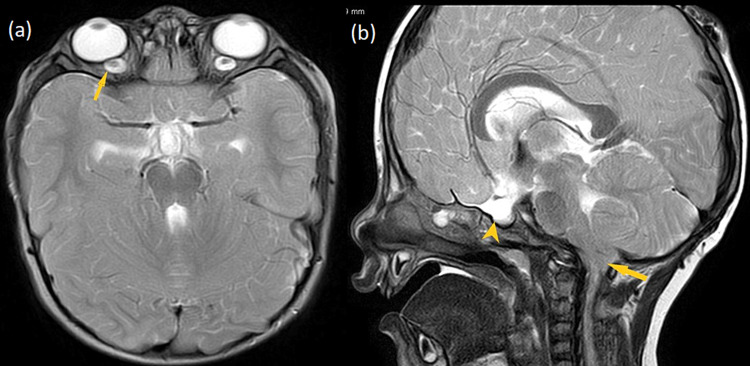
Axial (a) and sagittal (b) T2-weighted images. The images are showing prominent optic dural sleeves and flattening of optic discs (yellow arrow) (a), partially empty sella with widened diaphragma sellae (yellow arrowhead) and cerebellar tonsillar herniation (yellow arrow) (b). T2W: T2-weighted
